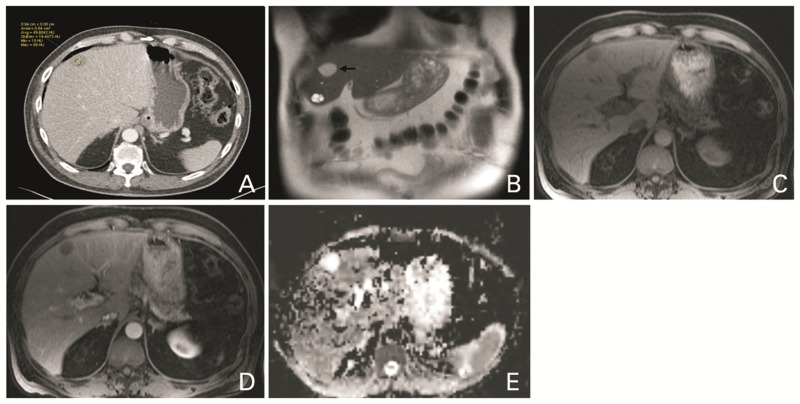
A 68-year-old man with ciliated hepatic foregut cyst. Contrast-enhanced CT (A) shows a hypodense lesion in segment 4B, with attenuation slightly more than water (49 Hounsfield Unit). The lesion (arrow) is bright on T2 WI (B) but not as bright as a cyst also seen in segment 4B. The lesion is hypointense on T1 weighted image (C) with no enhancement after contrast injection (D). There is no associated diffusion restriction, as can be seen on the ADC image (E).
